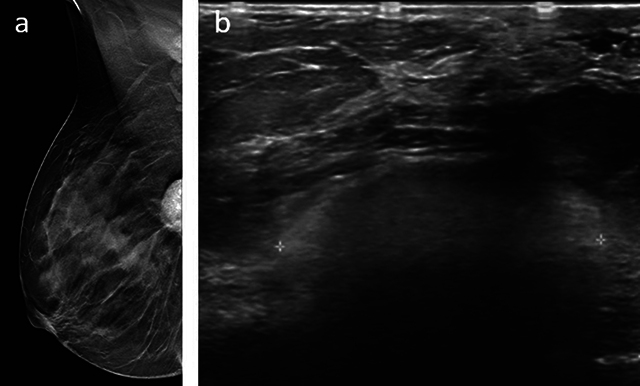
a. Right mammogram (mediolateral oblique view): partially visible oval, well-circumscribed deep mass. b. Ultrasonography of the right breast: deep, well-circumscribed hyperechogenic mass with typical “snowstorm.”
